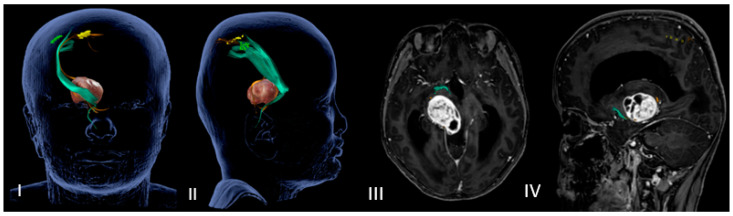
(I–IV) Neuronavigated transcranial magnetic stimulation pre-op study. (I,II) A 3D reconstruction of the nTMS data that shows cortical maps of the right spinal tract (CST) for the hand (green) and foot (yellow) and its relationship with the thalamopeduncular tumor of Case B. (III,IV): The brain MRI merged with the nTMS data shows that the cortical spinal tract (CST) runs antero-laterally with respect to a right thalamopeduncular tumor.
